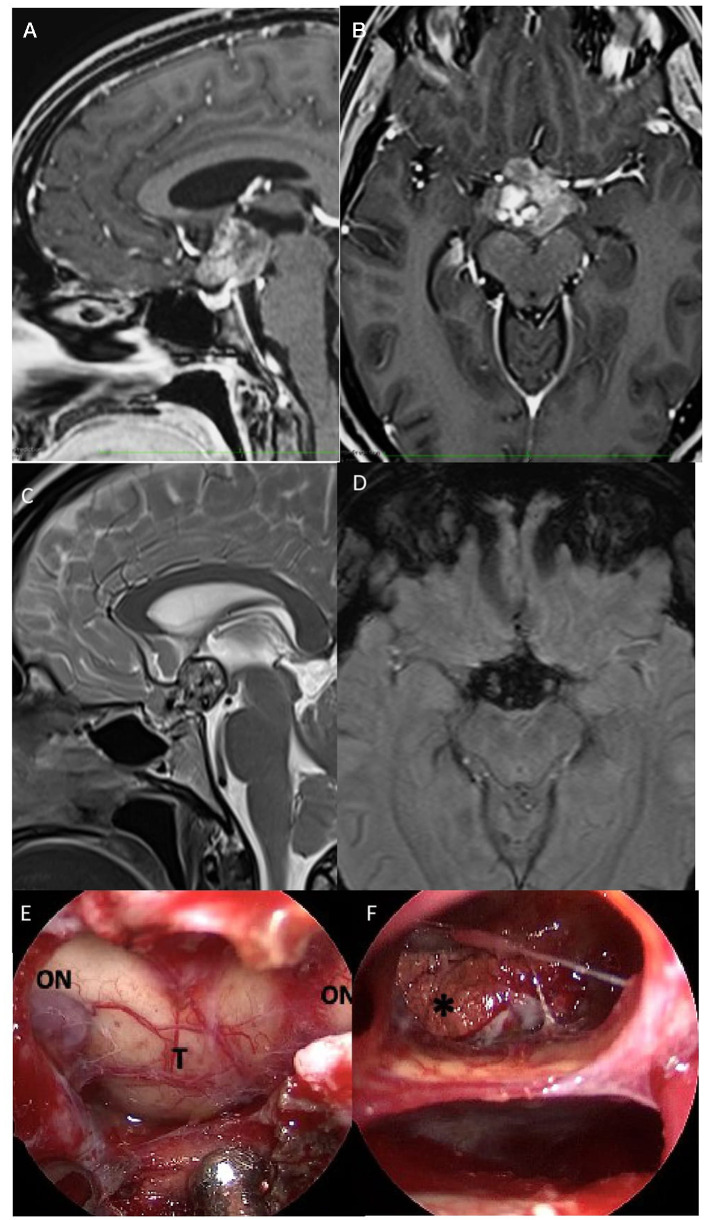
Preoperative sagittal (A) axial (B) post-gadolinium, and sagittal (C) T2 MRI scan demonstrated a suprasellar mass with heterogenous low signal in T1 images and an intermediate high signal in T2 images, with cystic and calcific components of the lesion. Axial (D) susceptibility-weighted imaging (SWI) showed signal dropout (hypointensity) in the sellar region. The lesion measured ~3 × 2,4 × 3, and 3 cm on anteroposterior, cephalocaudal, and transverse dimensions. (E) After dura opening, the evacuation of the intralesional hemorrhagic component localized inside the optic chiasm and infundibulum of the third ventricle was performed, which appeared dislocated below; (F) at the end of the procedure, a yellowish granulomatous formation was removed in fragments of dubious vascularization but suspected of a possible, already site of previous bleeding and adhering to the ventricular walls. ON, optic nerve; T, tumor. *Calcification.
